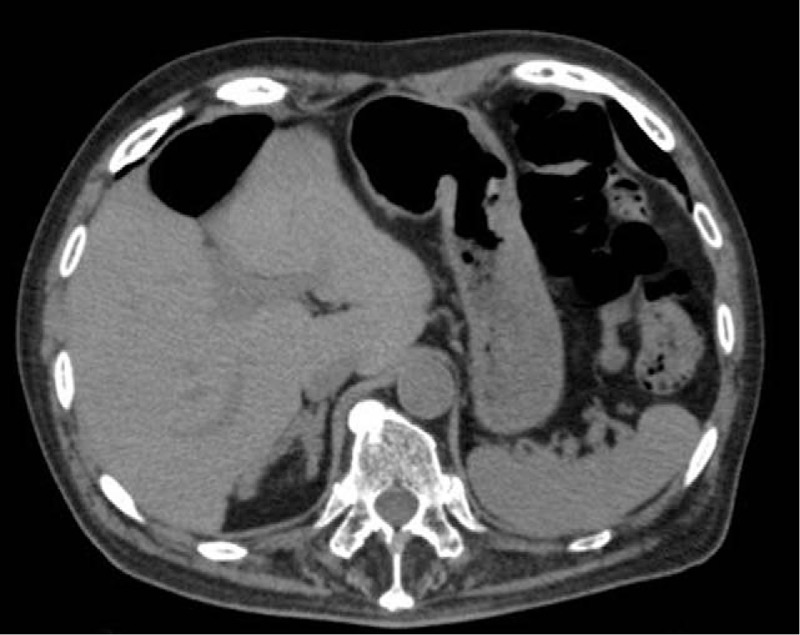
Plain abdominal computed tomography shows an almost normal-sized right adrenal gland and no residual left adrenal gland.
